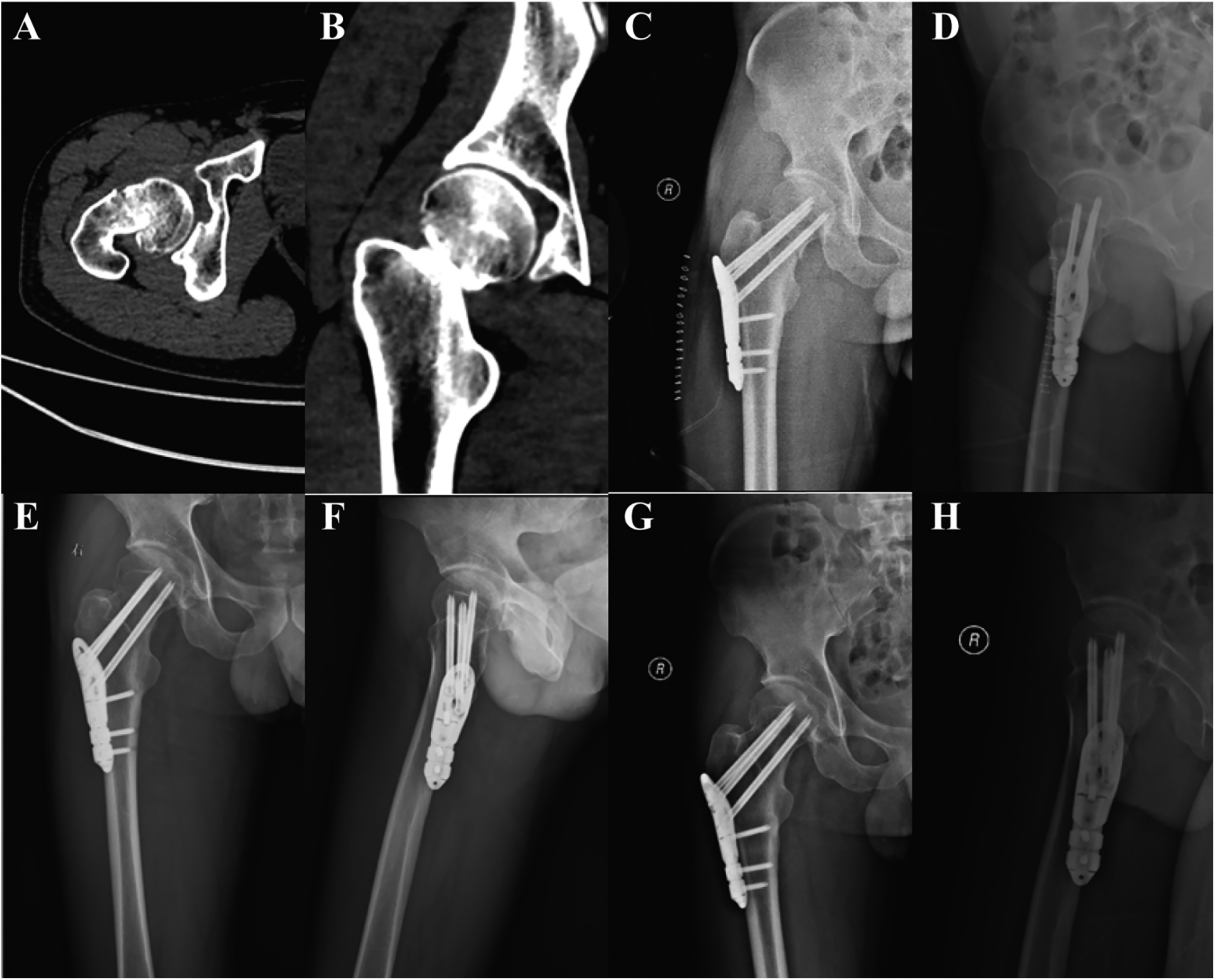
A 40-year-old male patient was admitted to the hospital due to pain and restricted range of motion in the right hip following a traffic injury, and he underwent PFLP treatment. (A,B) Pre-operative CT images of the patient showed a right femoral neck fracture, Garden type IV. (C,D) Review radiographs 1 day post-operatively in the anteroposterior and lateral positions showed good reduction. (E,F) Review radiographs 4 months post-operatively. (G,H) Review radiographs 12 months post-operatively.
